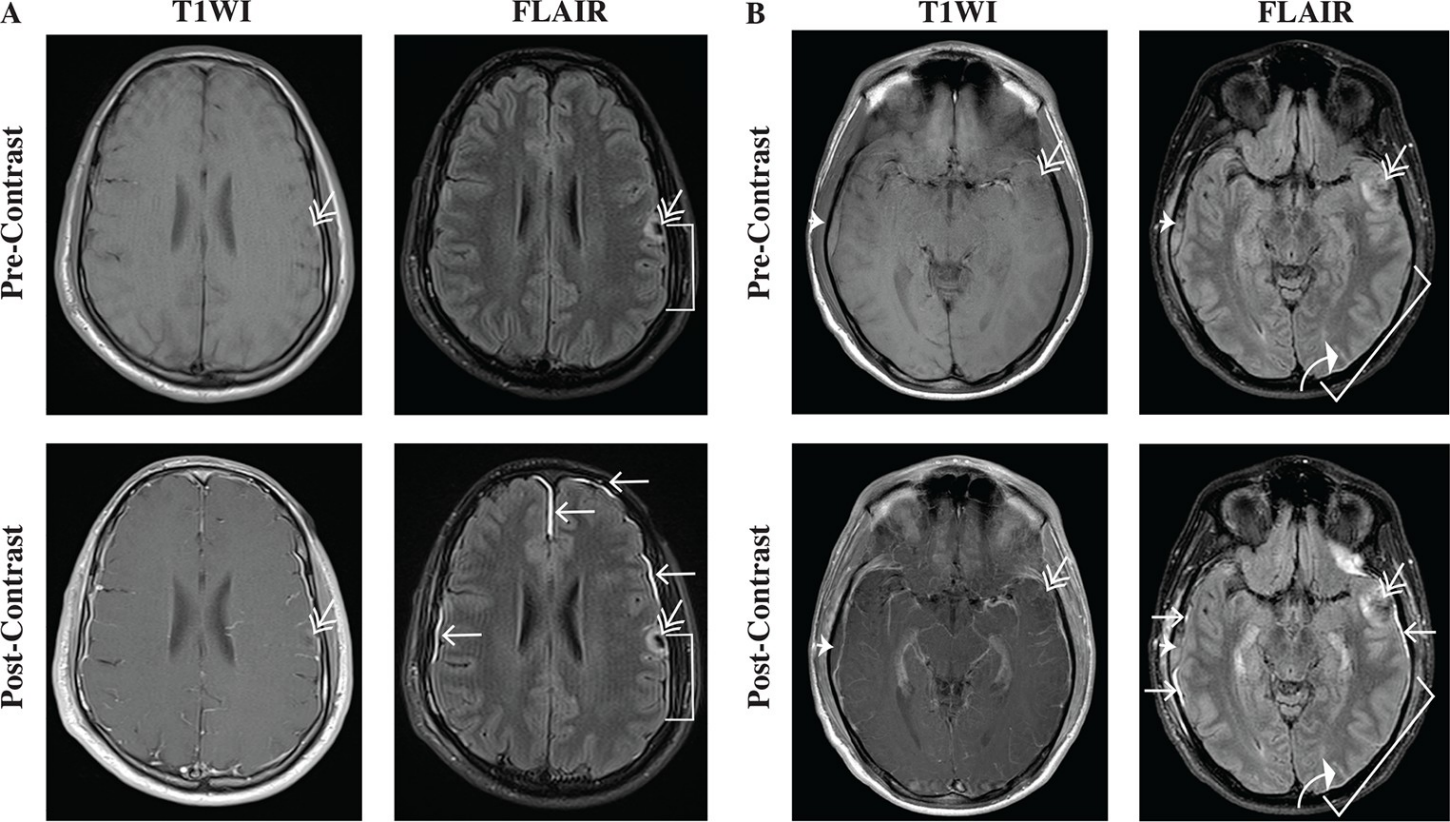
T1WI and FLAIR pre- and post-contrast MRI can reveal different types of TBI-related pathology in patients presenting acutely (< 48 hours) after injury. Radiological conventions are used here, with the patient’s left side on the right-hand side of the image. (A) The first series of images shows a representative example of a TBI patient with a contusion (double arrow), a subdural hematoma (bracket), and traumatic meningeal enhancement (TME) (single arrows). On both T1WI pre- and post-contrast images, the small contusion on the patient’s left side is more noticeable on FLAIR images secondary to associated edema. The pre-contrast and post-contrast FLAIR demonstrate a thin subdural hematoma adjacent to the contusion, which is not readily apparent on the T1WI images. On post-contrast FLAIR, areas of TME are also evident. In comparison, the T1WI post-contrast imaging reveals mostly vascular patterns in the parenchyma and along the meninges. (B) The second series of images shows images from another representative TBI patient with a small contusion (double arrow), a small epidural hematoma (thick arrowhead), a thin subdural hematoma (bracket), and associated subarachnoid hemorrhage (curved white arrow), in addition to TME (single arrows).
